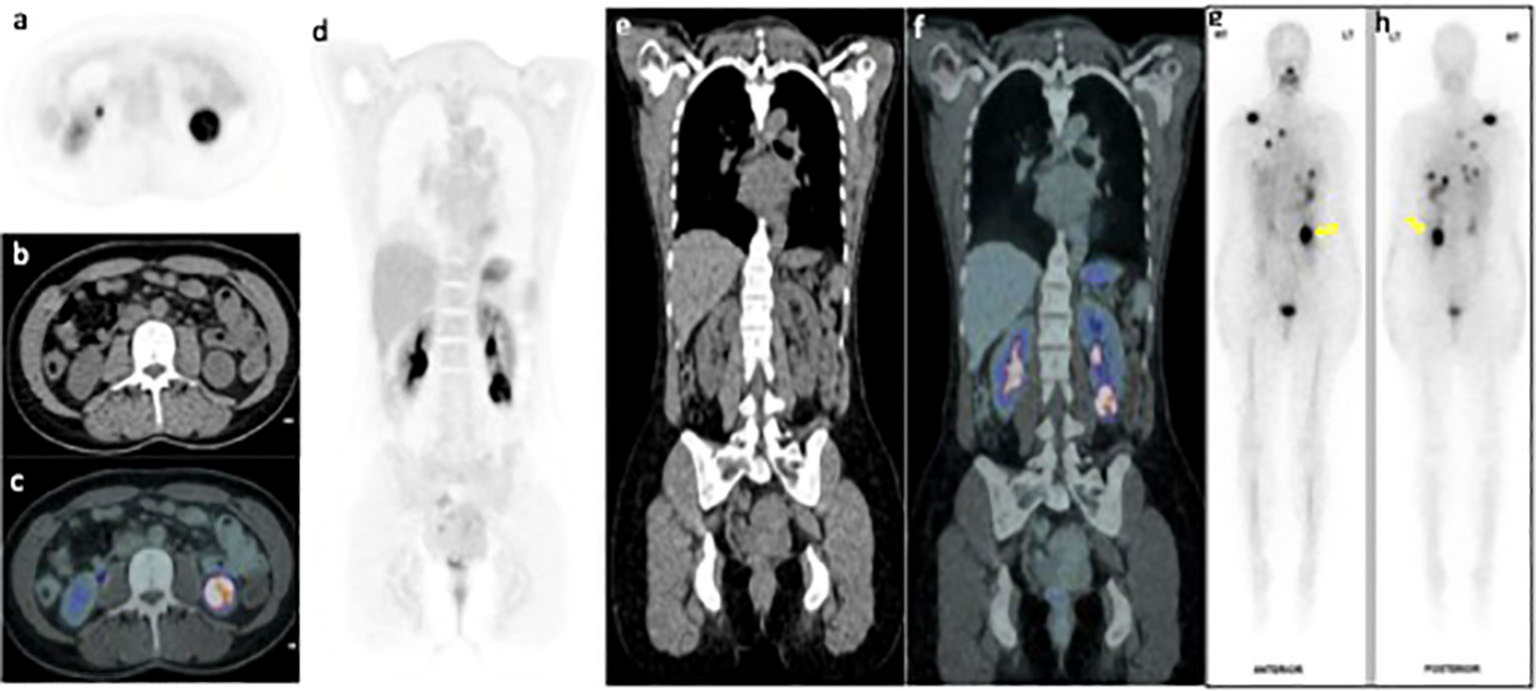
FDG PET/CT axial (A-C) and coronal (D-F) PET, CT and fused PET/CT images of the left lower pole renal mass (arrow) demonstrating interval increase in size and FDG uptake with SUVmax16.9. Whole body I^131^ post therapy anterior and posterior scan (G, H) performed within 6 weeks of the PET image, shows significant increase in I^131^ accumulation within the renal mass (yellow arrow).
